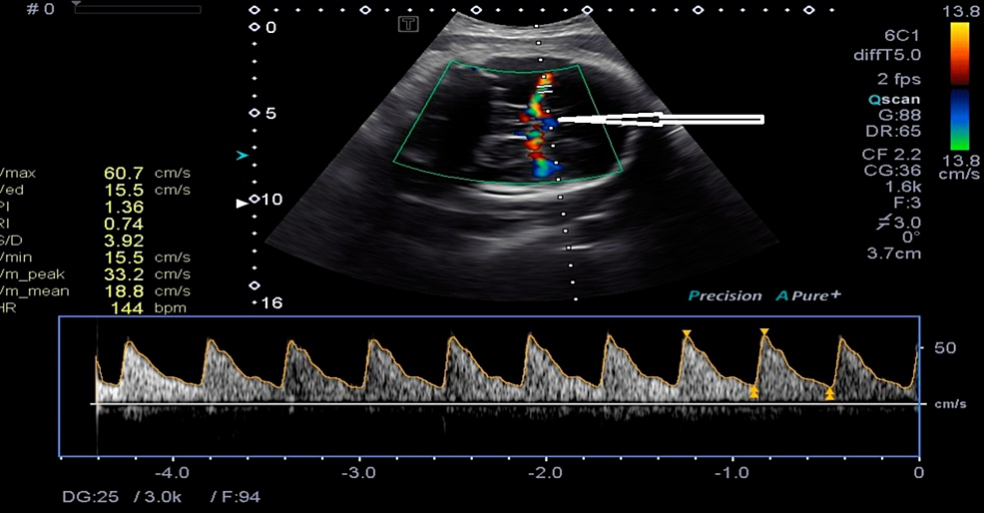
Doppler imaging of cerebral blood flow through MCA (arrow) showing marked changes at 37 weeks of GA in HIE. MCA: middle cerebral artery; HIE: hypoxic-ischemic encephalopathy; GA: gestational age.
